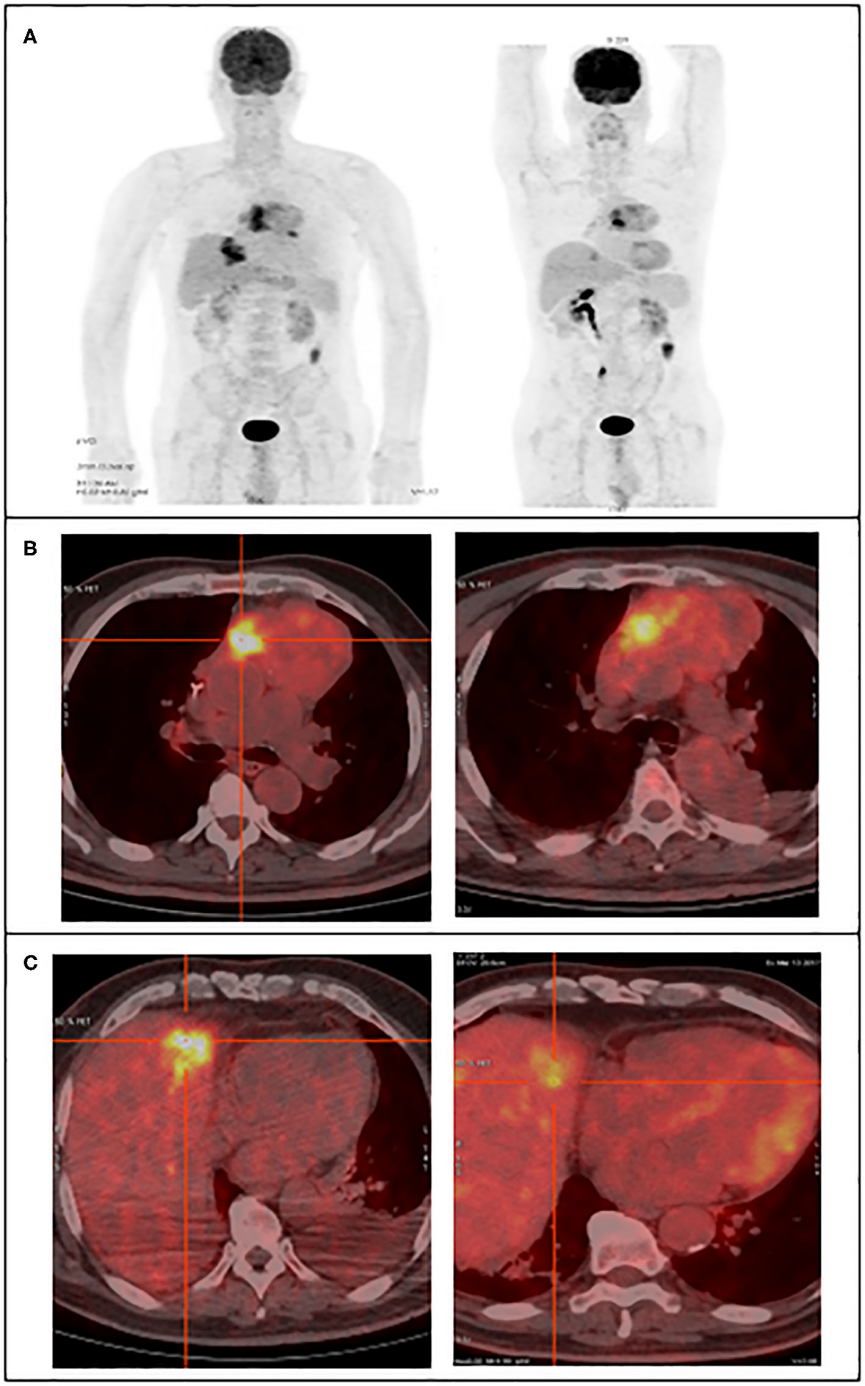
18F-FDG PET/CT images reveal the large hypermetabolic masses, in the superior mediastinum and the hepatic parenchyma, left figures before treatment and right figures post-treatment. (A) Maximum intensity projection image, (B) transaxial fusion image showing the mediastinal tumor, (C) transaxial fusion image showing the liver tumor.
